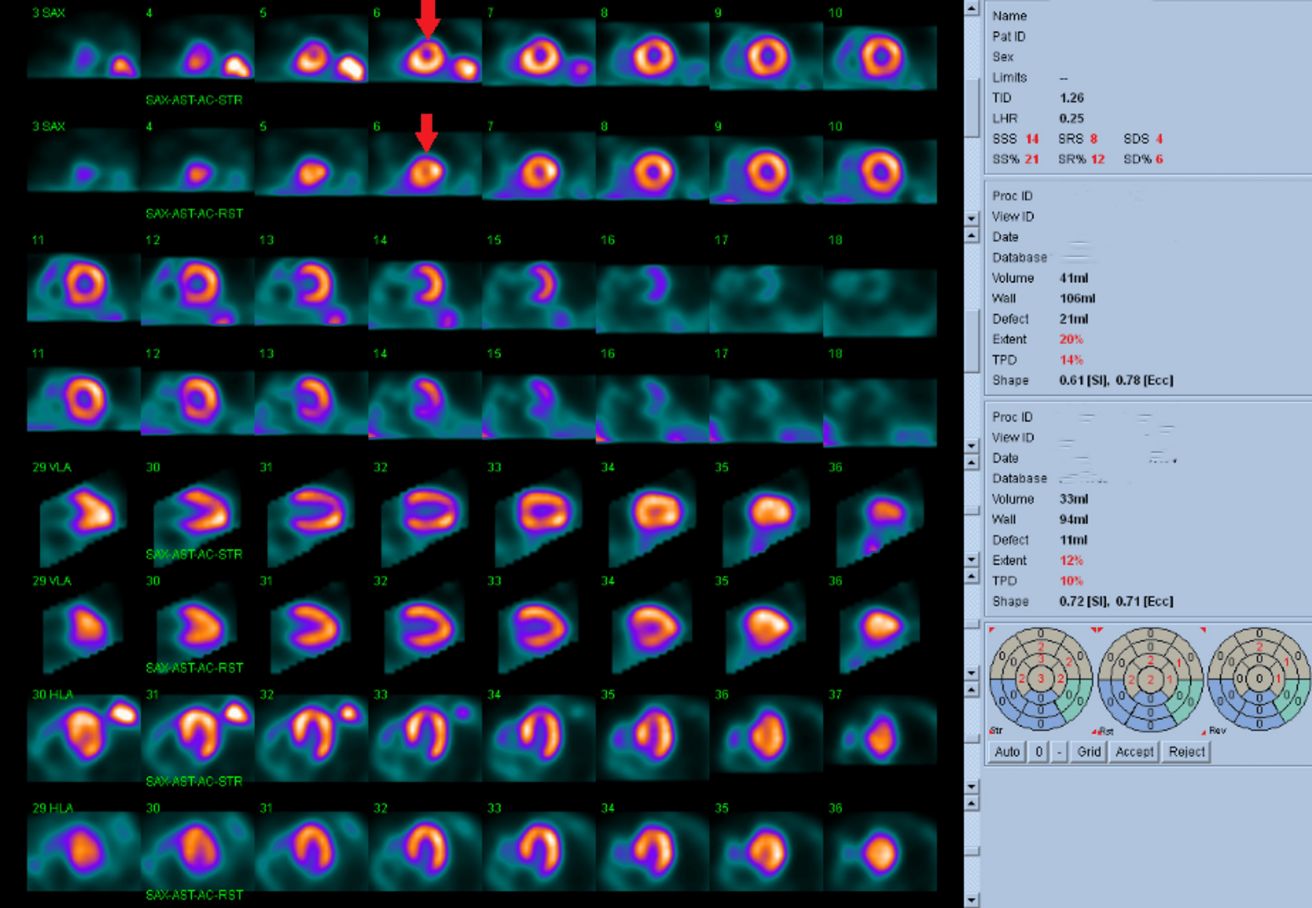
Myocardial perfusion SPECT demonstrating normal perfusion and hyperdynamic left ventricular function. Images demonstrate homogeneous tracer uptake without fixed or reversible perfusion defects, consistent with normal perfusion. Red arrowsindicate the markedly small left ventricular cavity at stress and rest, consistent with hyperdynamic function. Quantitative analysis confirmed a left ventricular ejection fraction >90%. SPECT: single-photon emission computerized tomography
